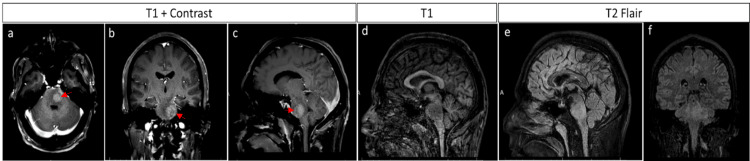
MRI Brain with contrast on the day of admission showing a heterogenous lesion on the left pons surrounded by vasogenic edema. The lesion is heterogeneously enhancing with contrast (a) + (b) + (c) (red arrow). The lesion appears hypointense in T1 (d) and hyperintense on T2 (e) + (f). FLAIR: Fluid-Attenuated Inversion Recovery.
